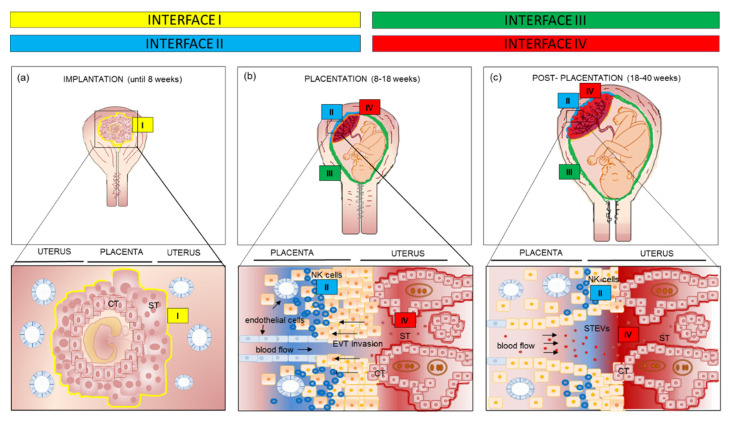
Main immunological interfaces between fetus and mother according to different gestational ages, from implantation to placentation process and post-placentation period. (a) Until 8 weeks of gestation the interface I is represented by ST invading the decidua; (b) in the second trimester of pregnancy the interface I is replaced by the interface II (decidual natural killer cells—extravillous trophoblast), III (decidua parietalis—amniochorion) and IV (maternal blood—ST); (c) in the third trimester interface IV is the dominant one. CT: cytotrophoblast; ST: syncytiotrophoblast; EVT: extravillous trophoblast.
